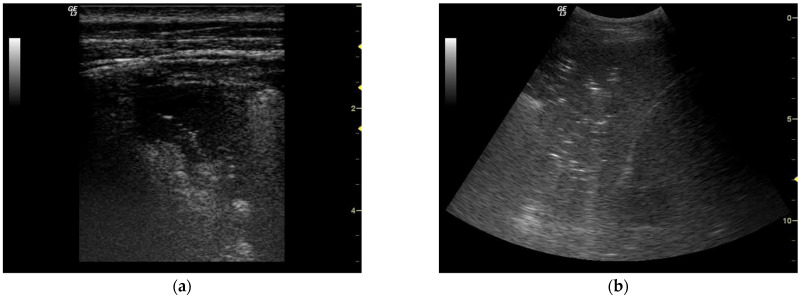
Lung ultrasounds: (a) Linear probe. Left superior lobe anterior segment of the lung. Subpleural lobar consolidation with irregular margins; the area of consolidation includes sonographic air bronchogram. (b) Curvilinear probe. Right posterior lower lobe posterior basal segment of the lung. Image of tissue echogenicity associated with aerial bronchogram (represented by hyperechoic punctuate images) strongly suggestive for alveolar syndrome.
